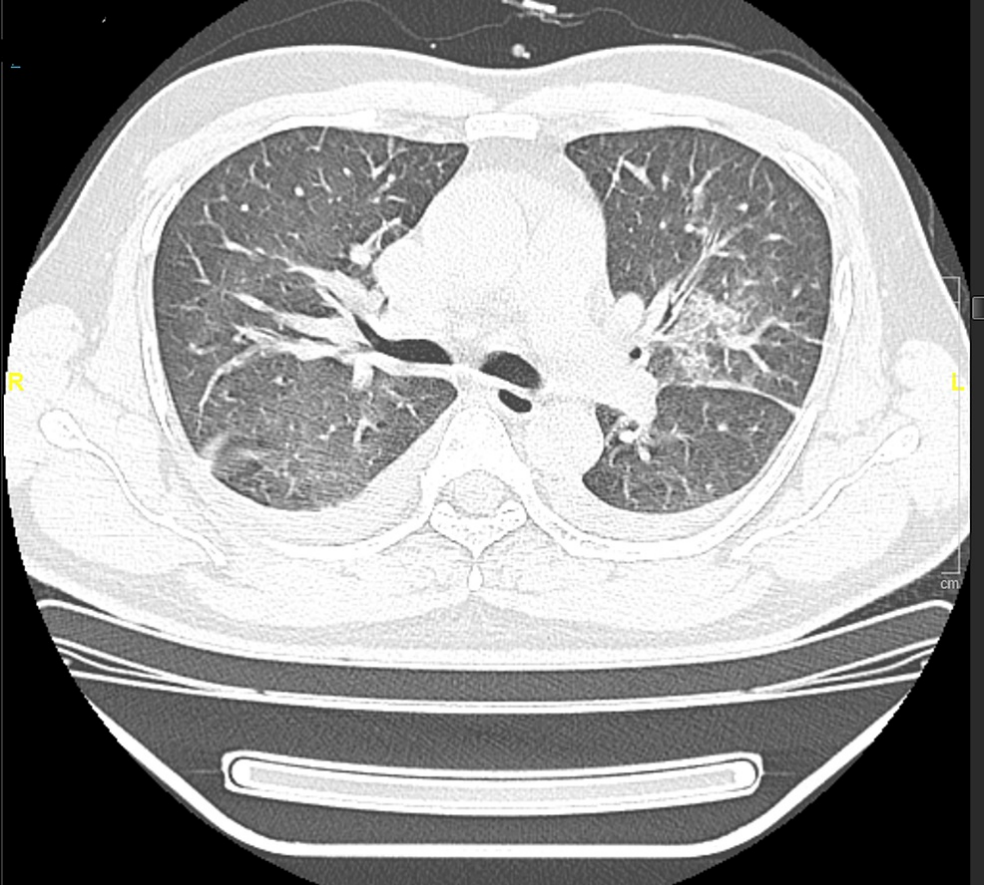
CT chest on the day of admission showing bilateral few small-sized patchy areas of consolidation and ground-glass opacity.
